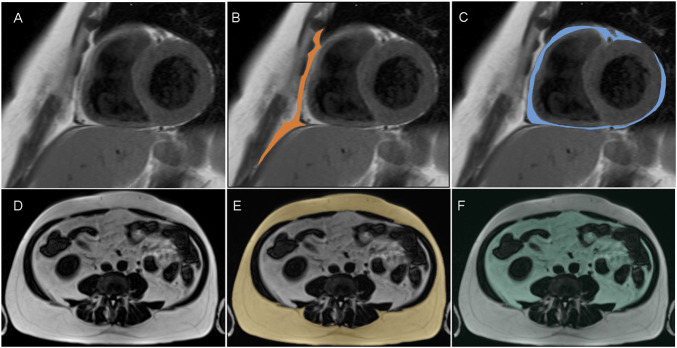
Segmentation of paracardial adipose tissue (PAT; B, colored) and epicardial adipose tissue (EAT; C, colored) from short axis dark blood t1-weighted turbo spin echo MR images (A; representative slice) and segmentation of subcutaneous adipose tissue (SAT; E; colored) and visceral adipose tissue (VAT; F; colored) from t1-weighted 3-D Volume Interpolated Breathold Examination (VIBE) Dixon MR images (D, presentative slice).
